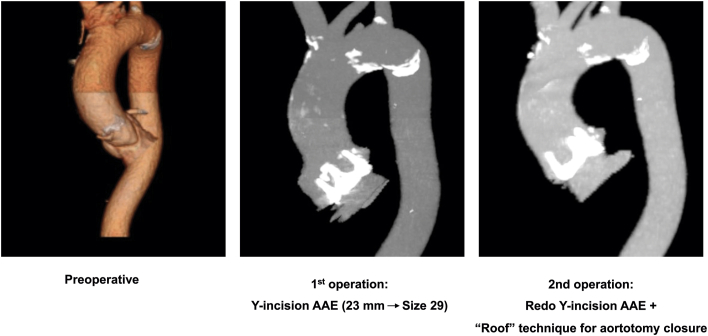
(Left) Preoperative, (middle) after post–index Y-incision aortic annular enlargement (AAE), and (right) postreoperation after Y-incision aortic annular enlargement computed tomographic angiography images. Postreoperation computed tomographic angiography demonstrates a well-seated size 29 valve with the “roof” technique used to close the aortotomy.
